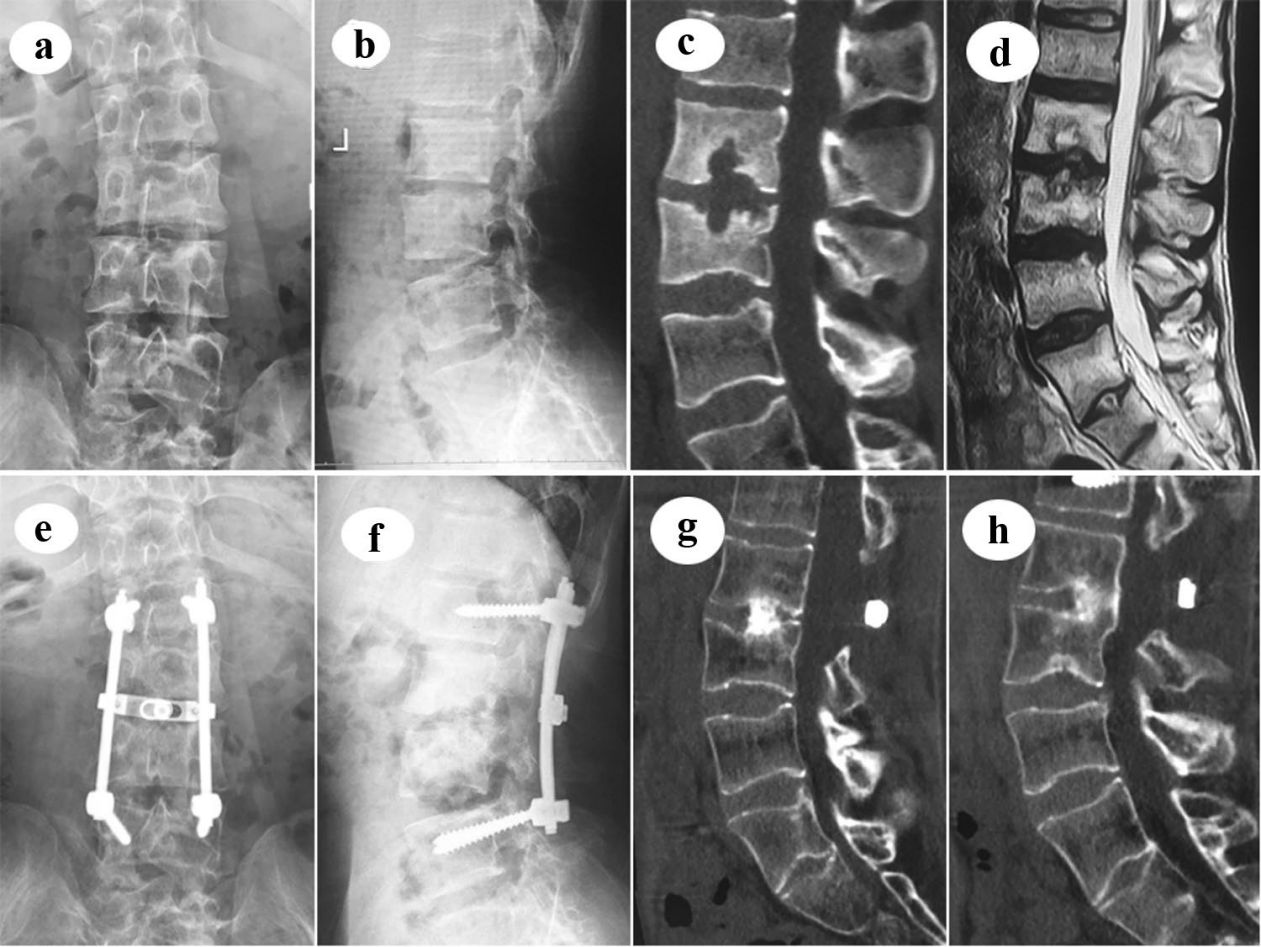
A 29-year-old male patient with L2–3 mono-segmental spinal TB, who complained of severe low back pain for 3 months, received single posterior debridement, interbody fusion and pedicle fixation. a, b Preoperative X-ray of AP and lateral images show roughly normal radiological presentation. c, d Preoperative CT and MRI images show the destruction of vertebral bodies and disc. e, f Postoperative X-ray of AP and lateral images show good position of pedicle screw fixation. g, h Postoperative CT images show solid interbody fusion had been achieved at the postoperative 24th month
